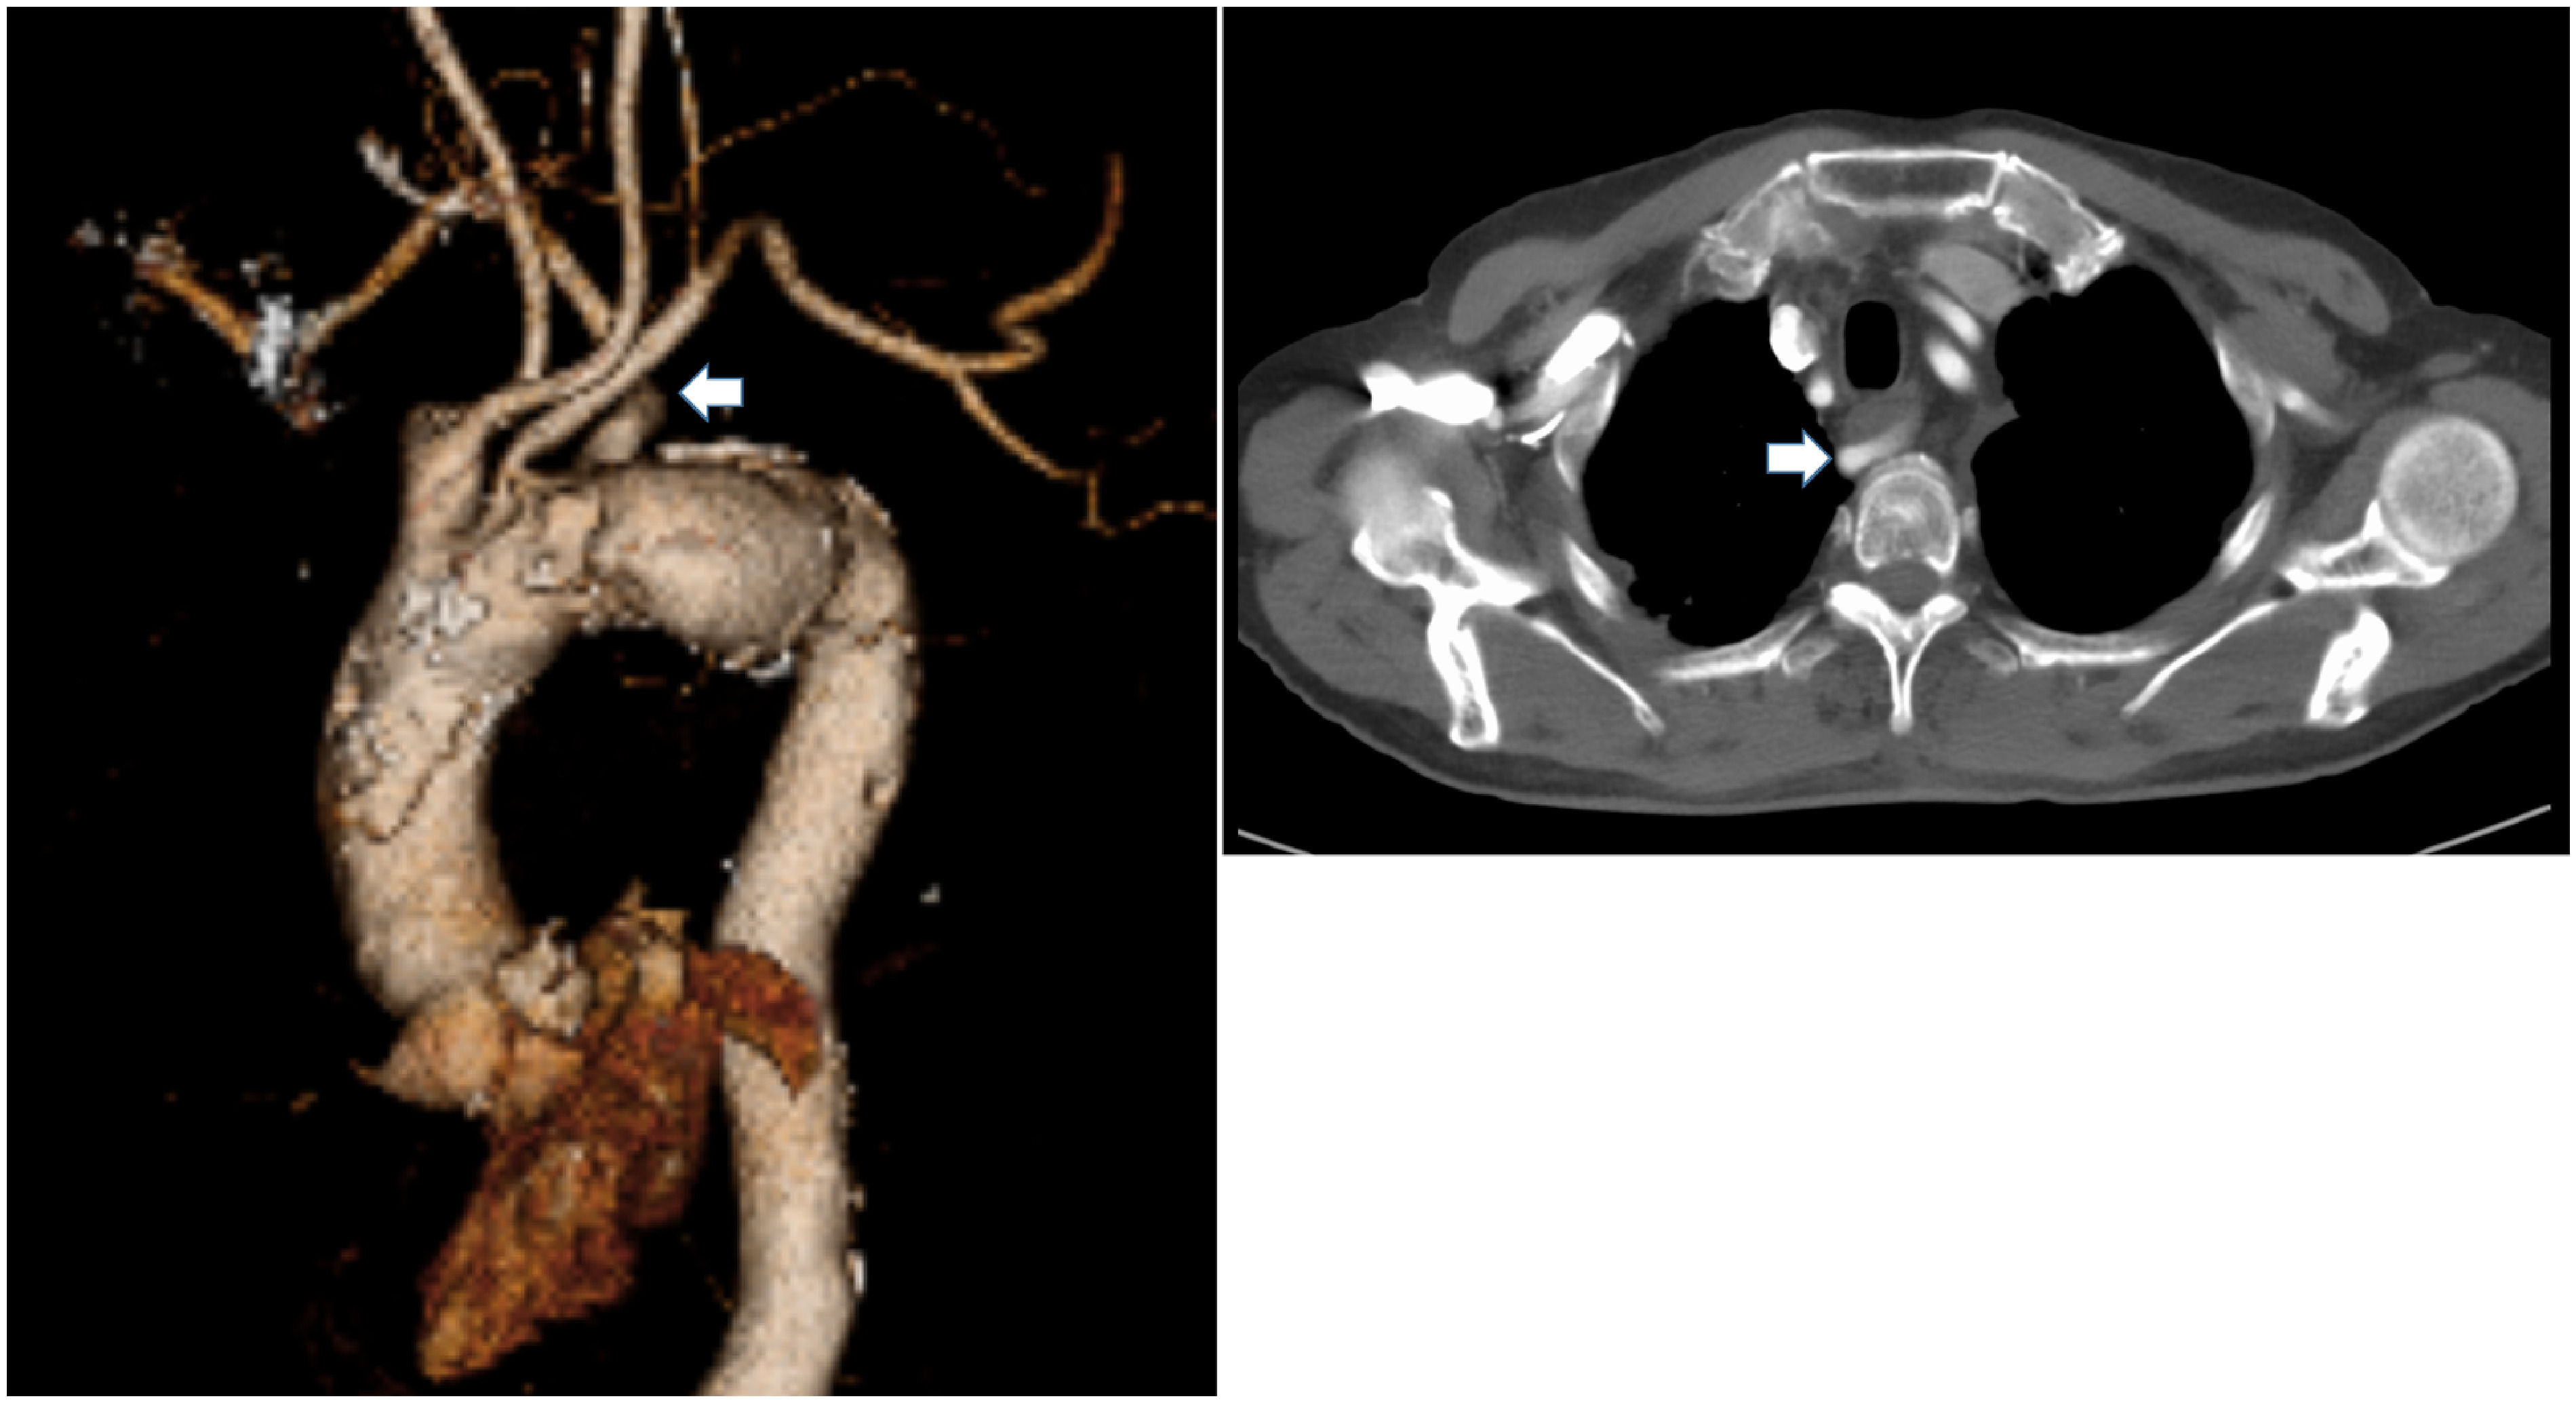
Fig. 1 Preoperative computed tomographic angiography in the left anterior oblique view presented a fusiform-type aneurysm in the distal aortic arch, a common carotid trunk, and an aberrant right subclavian artery (arrow) originating from the back of the aortic arch (left). The axial view showed an aberrant right subclavian artery passing behind the esophagus and trachea (right).
